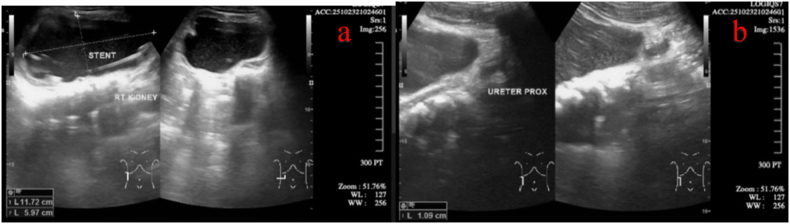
Postoperative abdominal ultrasonography showed that the (a) right renal pelvis was still dilated (b) without any hydroureter in the right ureter.
